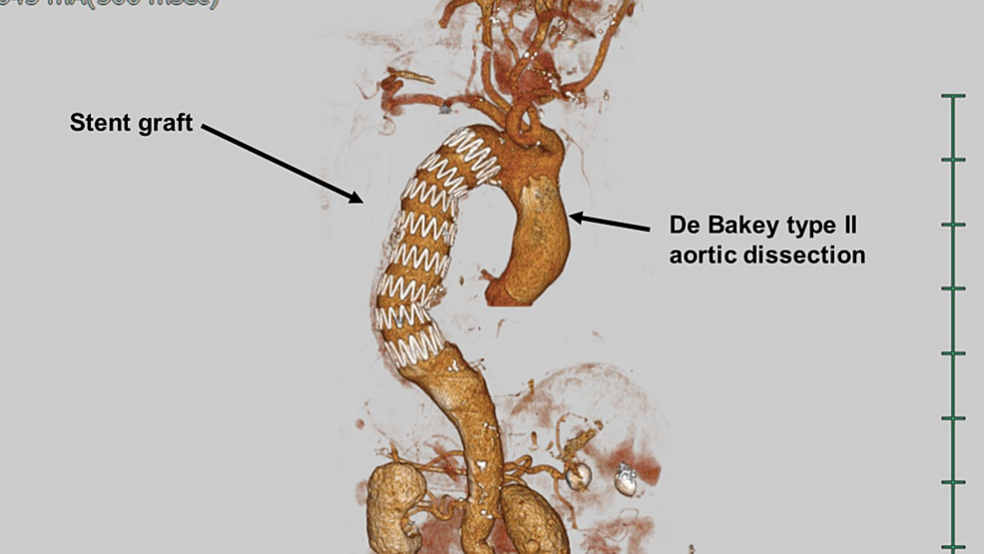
Preoperative computed tomography image A preoperative computed tomography scan showed no aortic dissection at the aortic arch.
